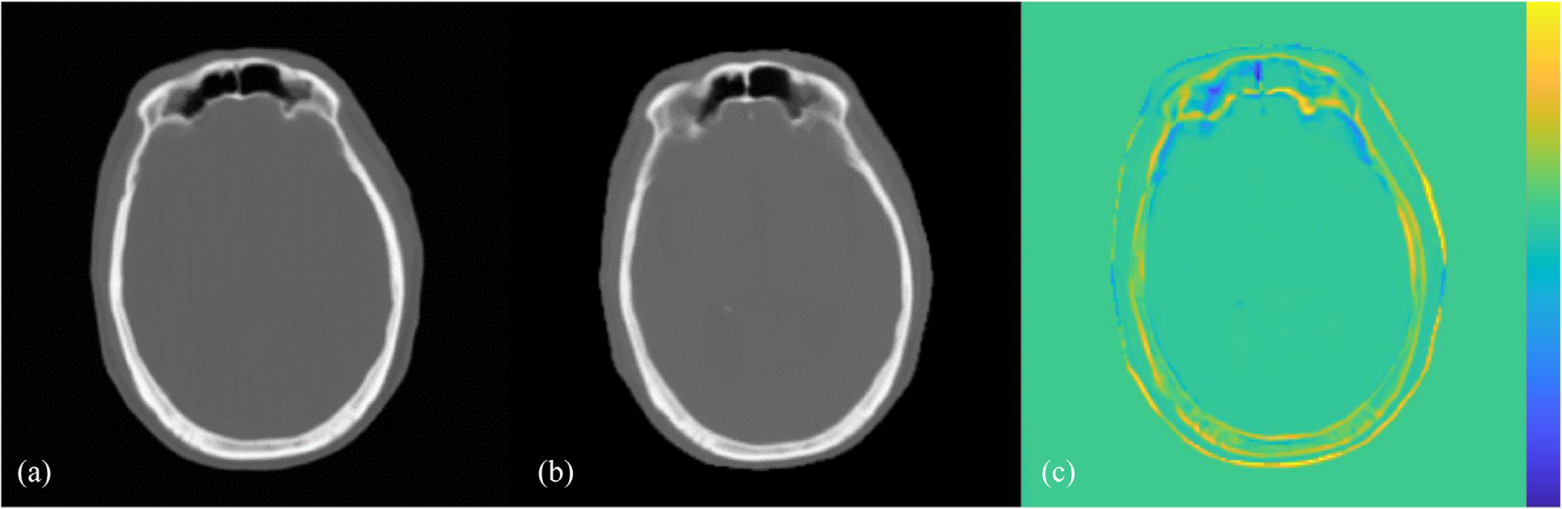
Axial image for subject with highest MAE among IMRT/VMAT samples. This demonstrated that area with high HU deviation were locating as bony interfaces surrounding with tissues and air. The Resampled MRCAT (a) was subtracted with planning CT (b) to obtain subtraction map (c) with yellow and blue colour contributed from resampled MRCAT and planning CT, respectively.
